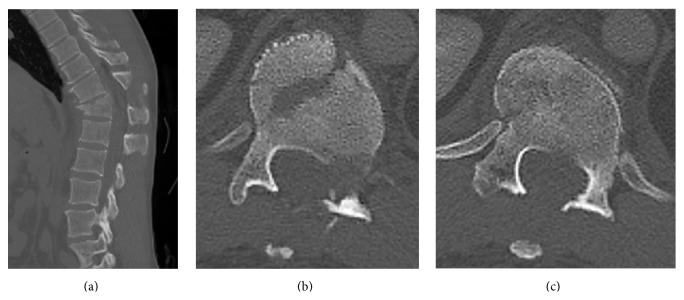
Preoperative computed tomography (CT) images of the spine. (a) Sagittal image, (b) axial image of upper T12, and (c) axial image of lower T12. Anterior, posterior wall, and left pedicle fractures of the T12 vertebra, spinous process fracture of T11, and right T11-12 facet dislocation.
